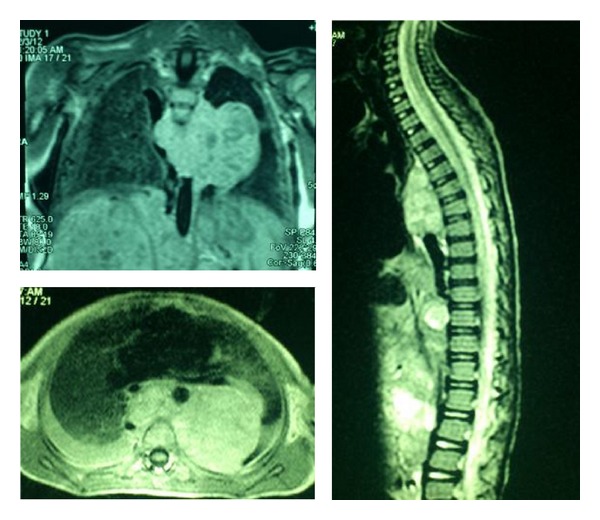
MRI showing left paravertebral mediastinal mass without intracanal invasion.
